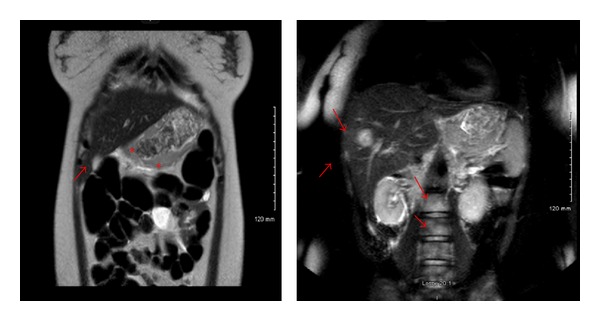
MRI with and without contrast of the abdomen showing at least two liver metastases, stomach thickening, and spinal metastasis.
